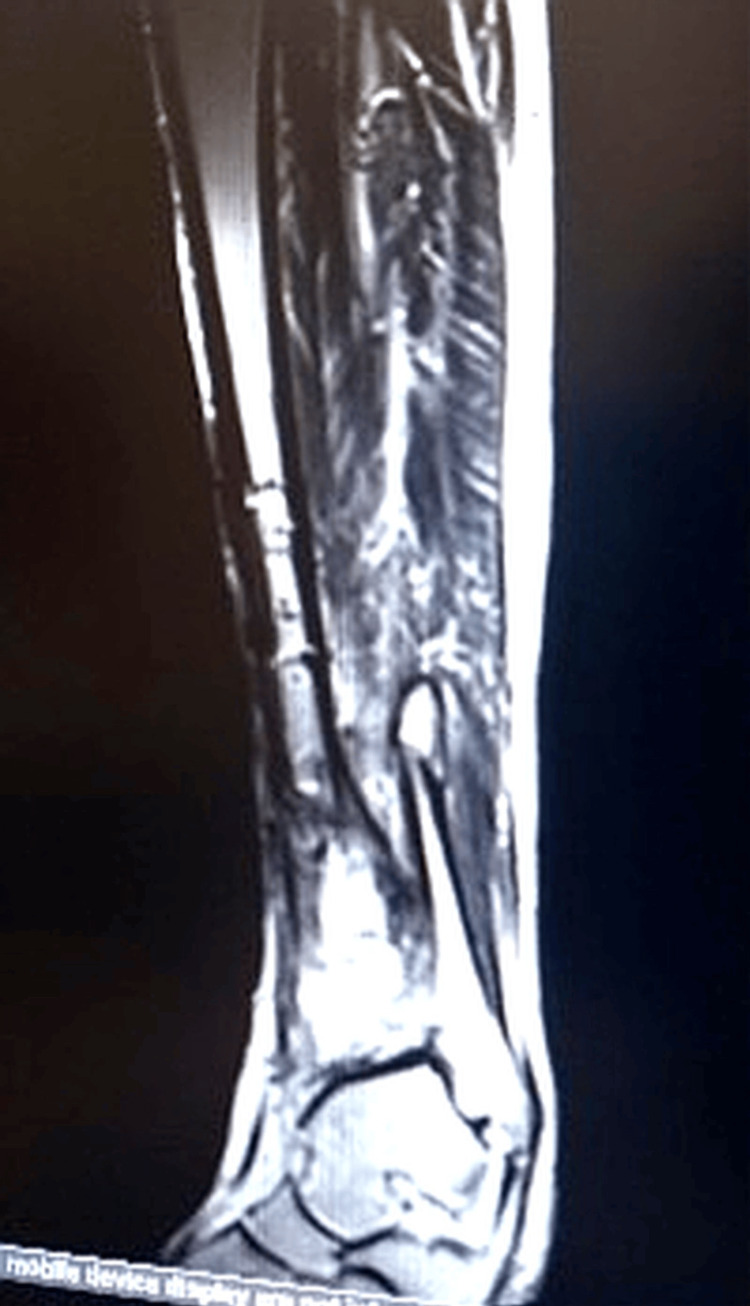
MRI demonstrating osteomyelitis with sequestrum
